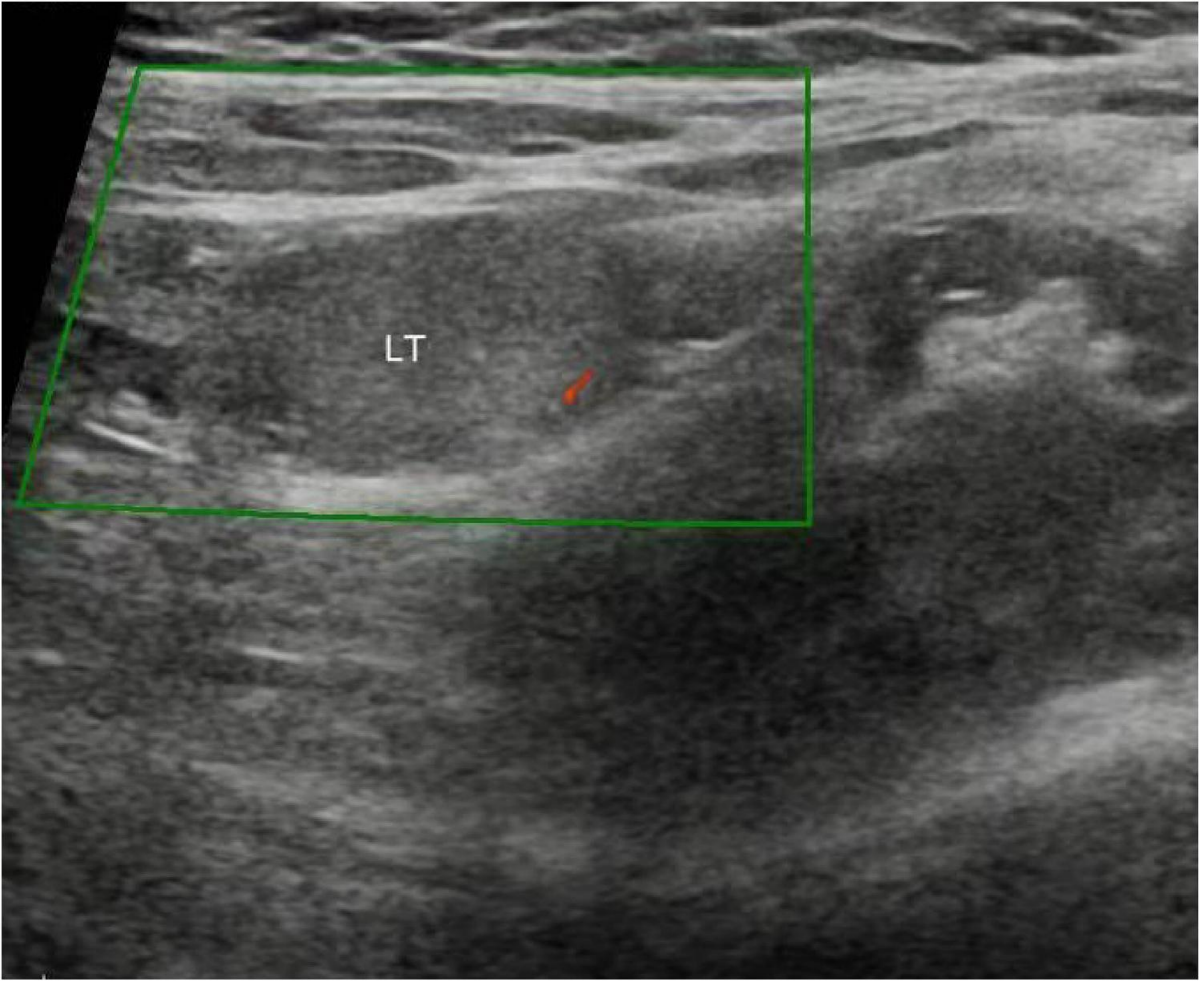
Preoperative B-ultrasound showed that the left testicle was situated in the abdominal cavity (green box).
